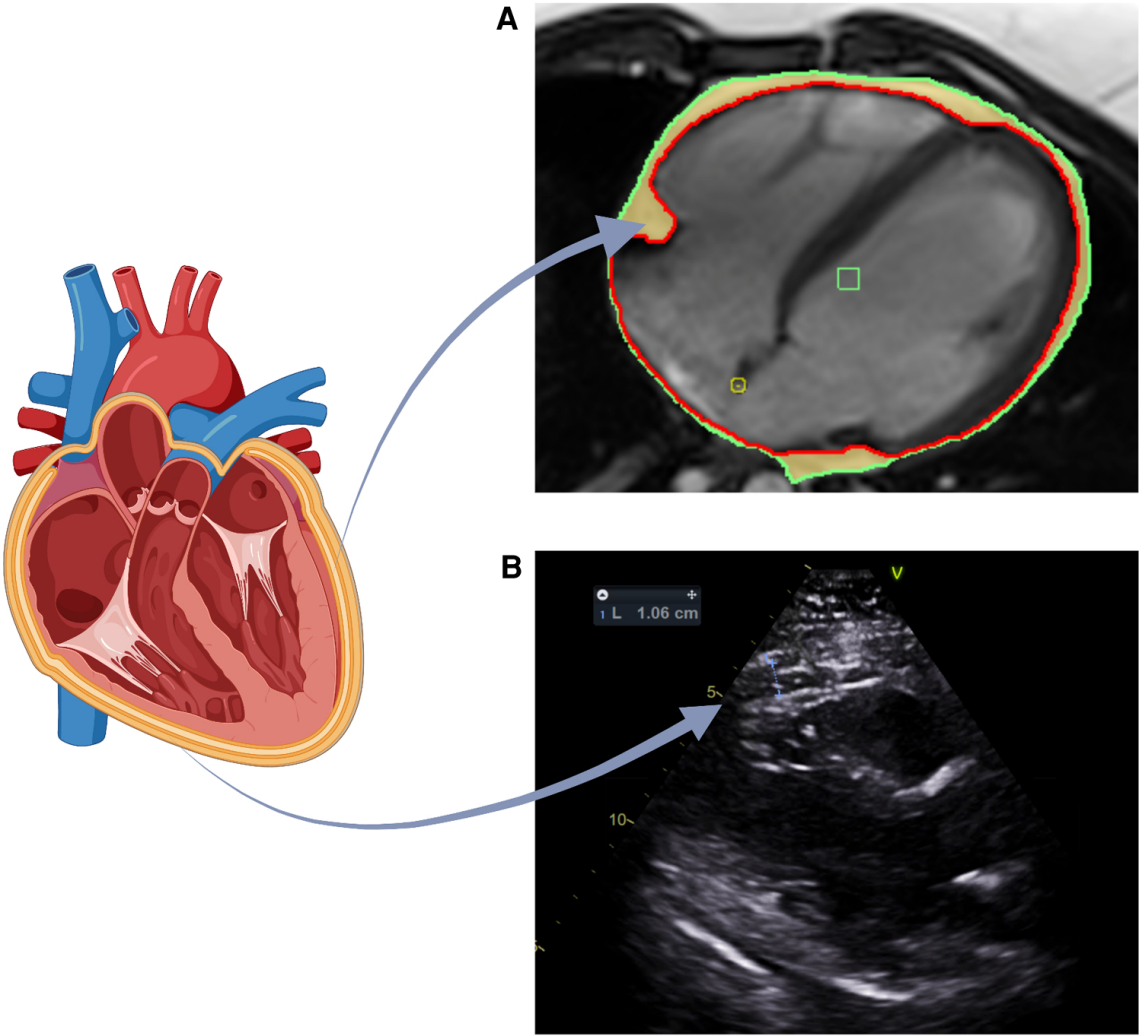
Assessment of EAT utilizing TTE and CMR (A) EAT volume assessed by CMR. EAT is defined as the adipose tissue between epicardium and pericardium. Long axis 4-chamber view: red line marks the epicardium, green line marks the pericardium and highlighted in yellow is the EAT. (B) EAT volume assessed by TTE. Parasternal long axis view used to measure EAT thickness anterior to the right ventricular free wall. The images shown for each technique were acquired from our institute (St Vincent's Hospital Sydney, Australia) with ethics approval.
